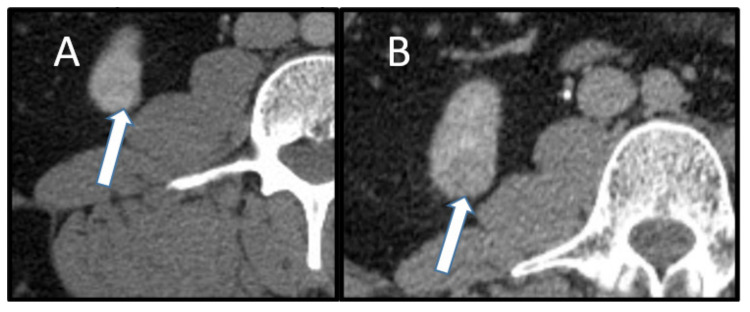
A 39-year-old man with a history of Cowden syndrome, follicular thyroid cancer, and lung cancer. Axial contrast-enhanced computed tomography demonstrates (A) corticomedullary phase with subtly enhancing 1.9 cm lesion (arrow) in the inferior right kidney, and (B) delayed imaging shows washout (arrow). This was pathologically proven to represent clear cell renal carcinoma. An additional 1.6 cm biopsy-proven clear cell renal cell carcinoma with the same imaging features was present in the superior pole left kidney.
